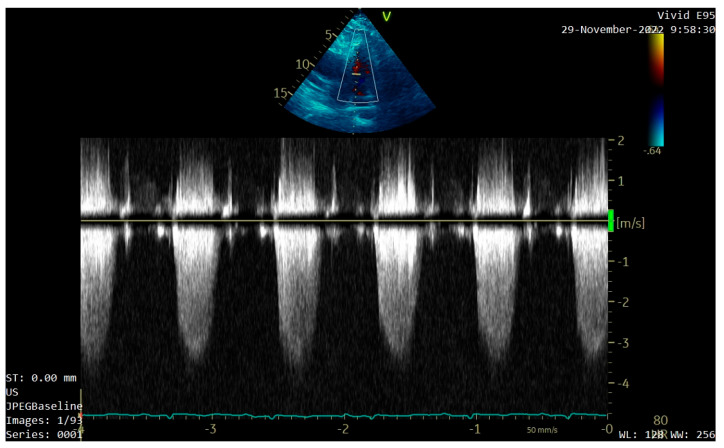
TTE Continuous Wave Doppler showing severe RVOT obstruction.
